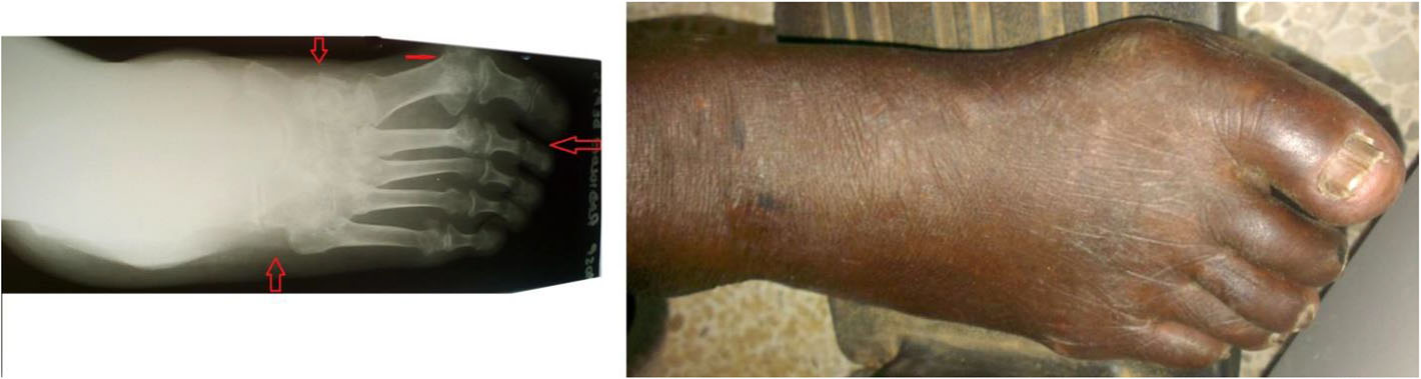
The anteroposterior view foot radiograph and the foot picture of the same patient with CA. The anatomical classification according to Sanders and Frykberg for this foot is type 1 (lesions in forefoot) and 3 (lesions in the tarsal bones). The modified Eichenholtz radiological classification for this foot is stage 3 (callus, bone remodelling at tarsus and metatarsophalangeal joints)
